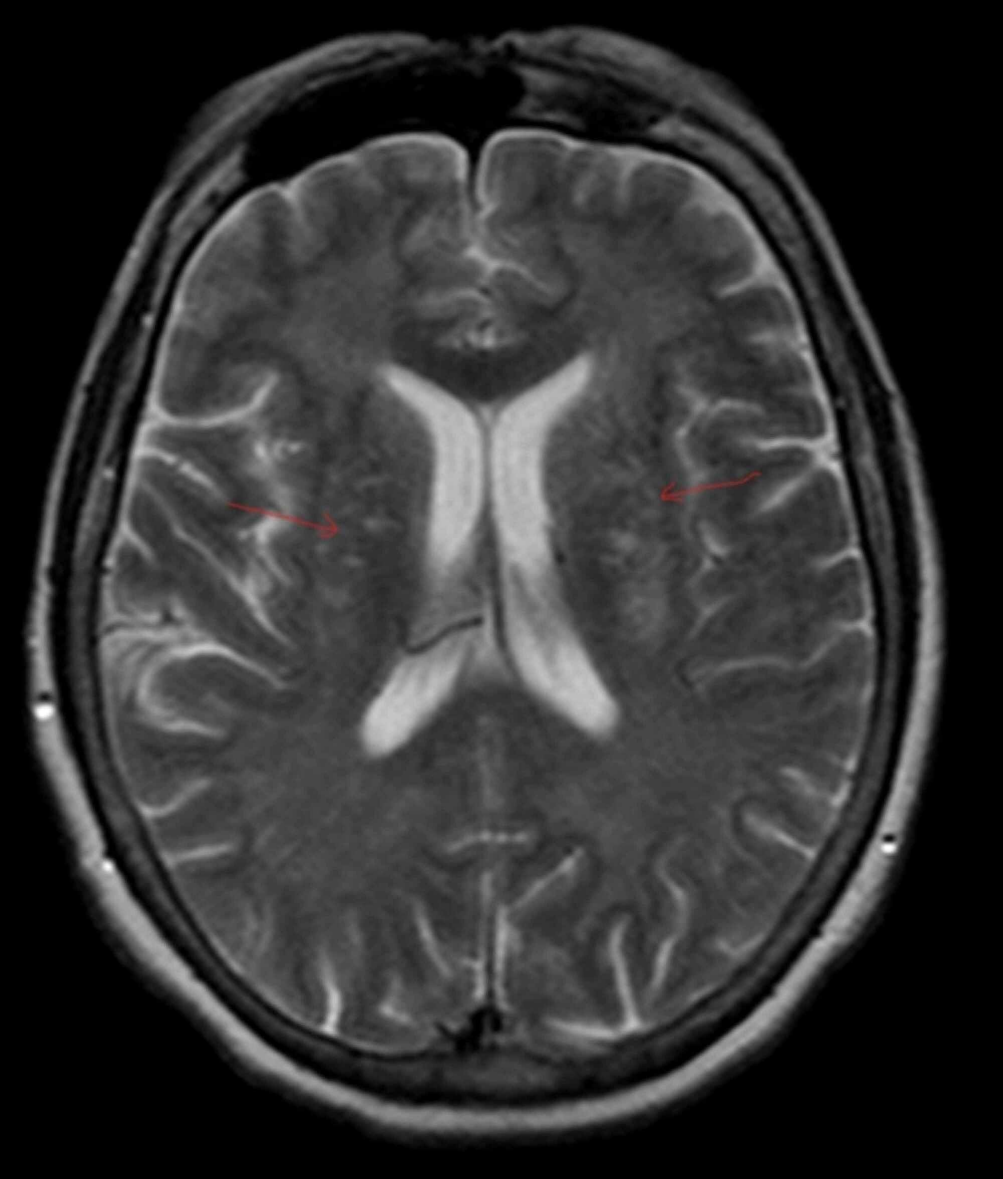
T2 weighted Axial MRI of the brain showing diffuse symmetric white matter hyperintensities in different parts of the brain
